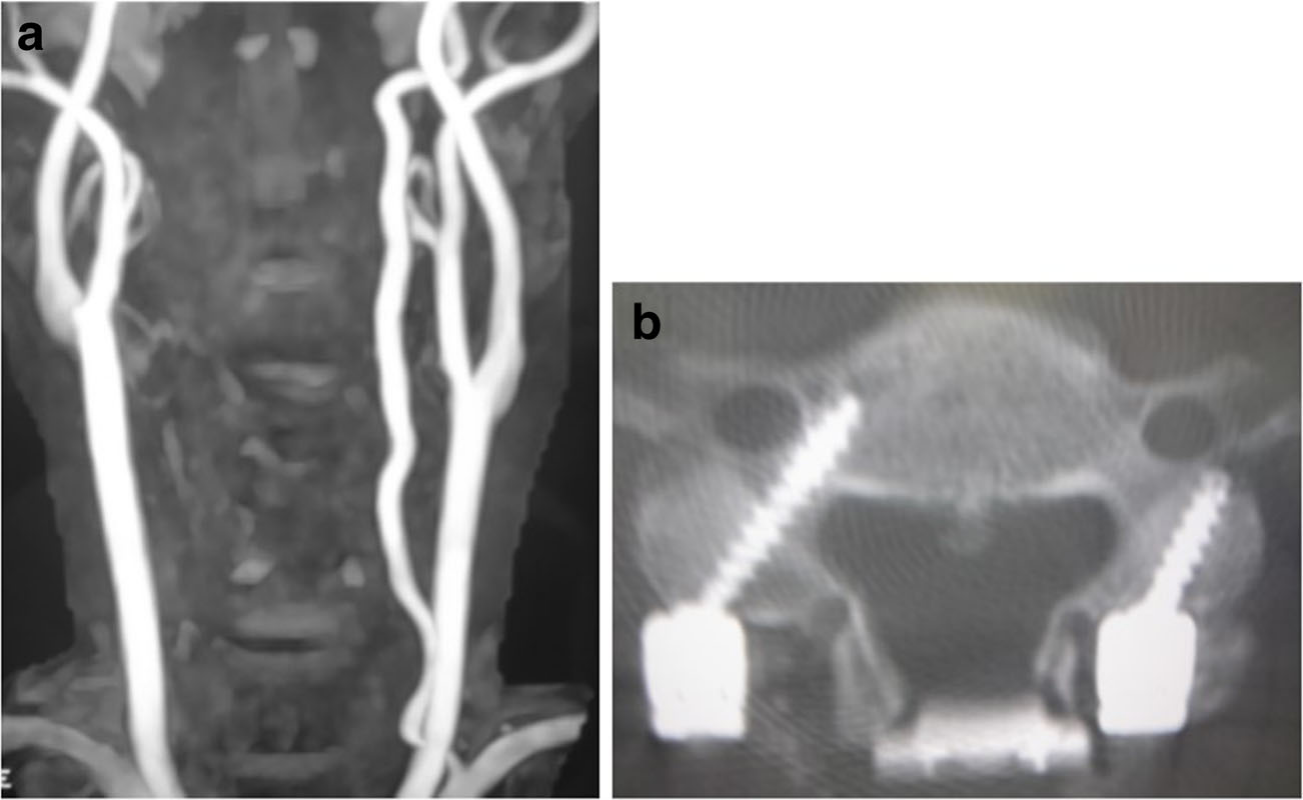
a Selection of cervical pedicle screw (CPS) and lateral mass screw (LMS) in cases in which preoperative MR angiography shows occlusion of the vertebral artery on the right side. b Postoperative CT shows that CPS was placed on the right side and the LMS on the left side
